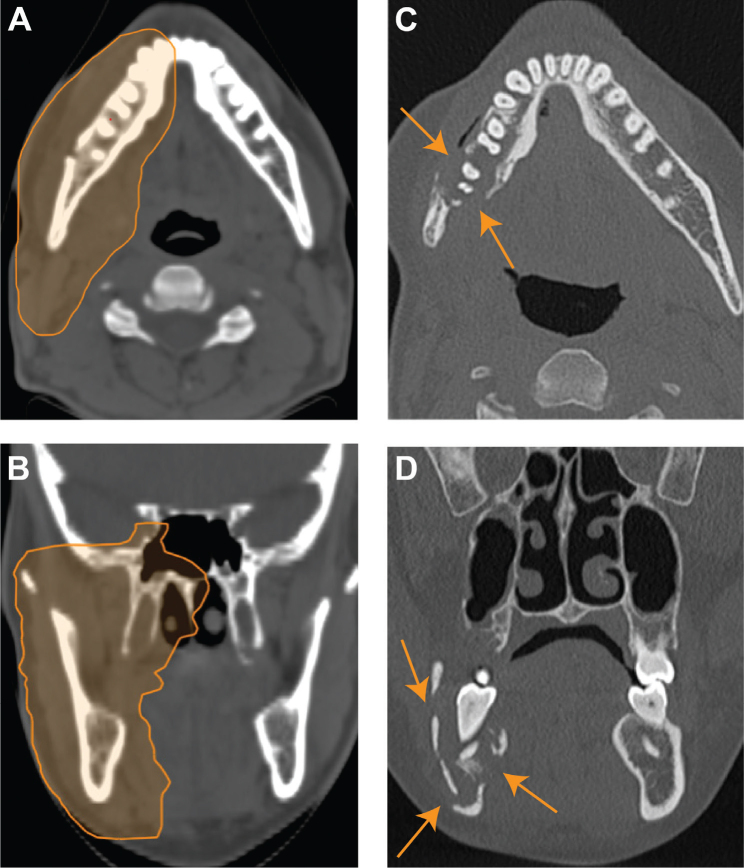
Carbon-ion radiotherapy (CIRT) patient no. 5: Osteoradionecrosis (ORN) development: Axial (A) and coronal (B) views of the treatment plan with the planning target volume (PTV) prescribed 68.8 Gy (relative biological effectiveness [RBE]). Axial (C) and coronal (D) computed tomography (CT) scans show a lucent area with trabecular loss, bicortical erosion, and pathological fracture (arrows). The findings are consistent with grade 3 ORN involving the right mandibular body and angle, which was diagnosed 18 months after CIRT.
